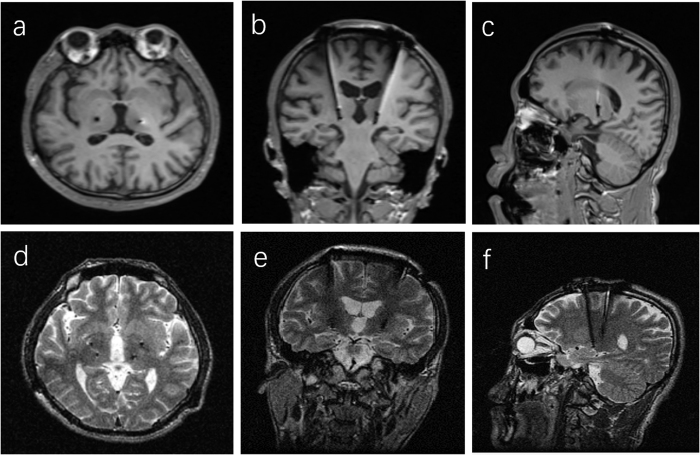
Representative imaging of DBS electrode localization in the MDS patient P1. (a–c) Postoperative T1-weighted MR images after initial Vim DBS. (d–f) Postoperative T2-weighted MR images after subsequent GPi DBS. a/d: axial plane, b/e: coronal plane; c/f: sagittal plane.
